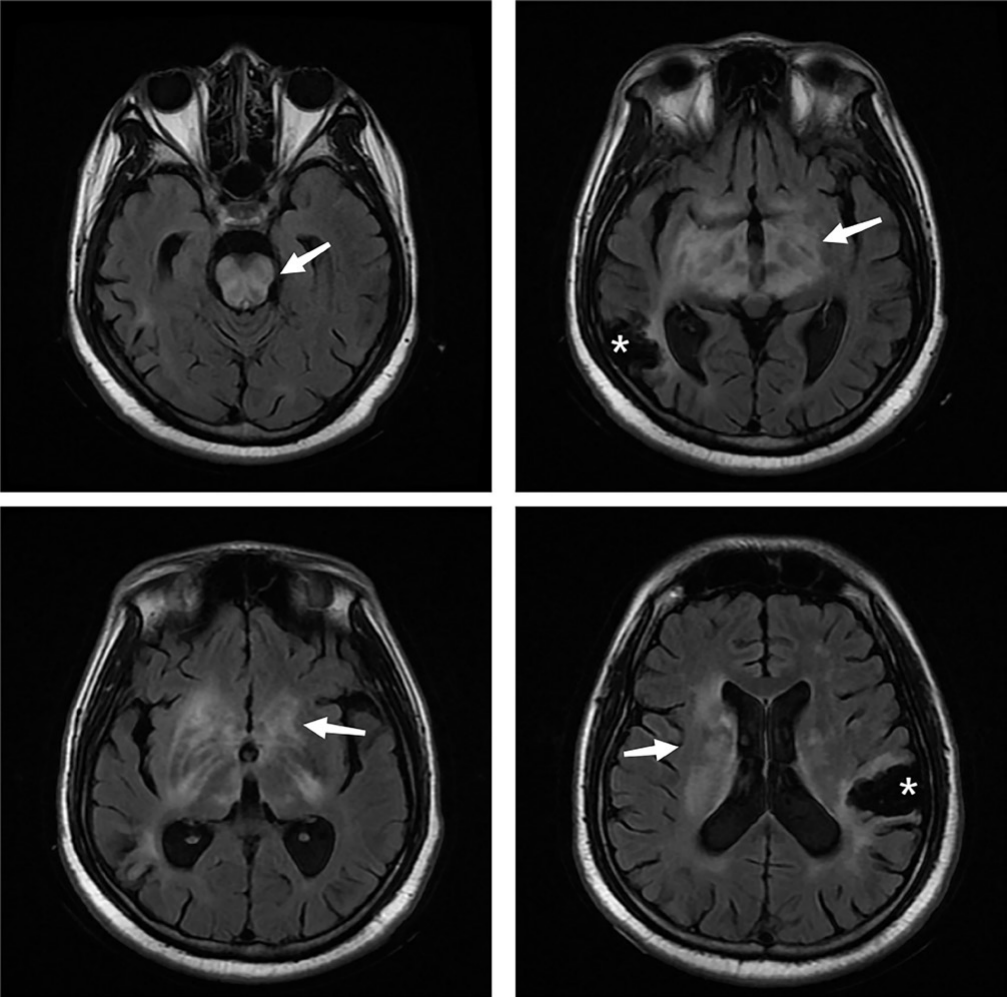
Cranial MRI before treatment: Diffuse abnormal signal in the pons, midbrain, basal ganglia, thalamus and beside the right lateral ventricle (arrows), softened lesions in the right temporal parietal occipital lobe, left frontal lobe and the left cerebellar hemisphere. *obsolete cerebral infarction. MRI, magnetic resonance imaging.
